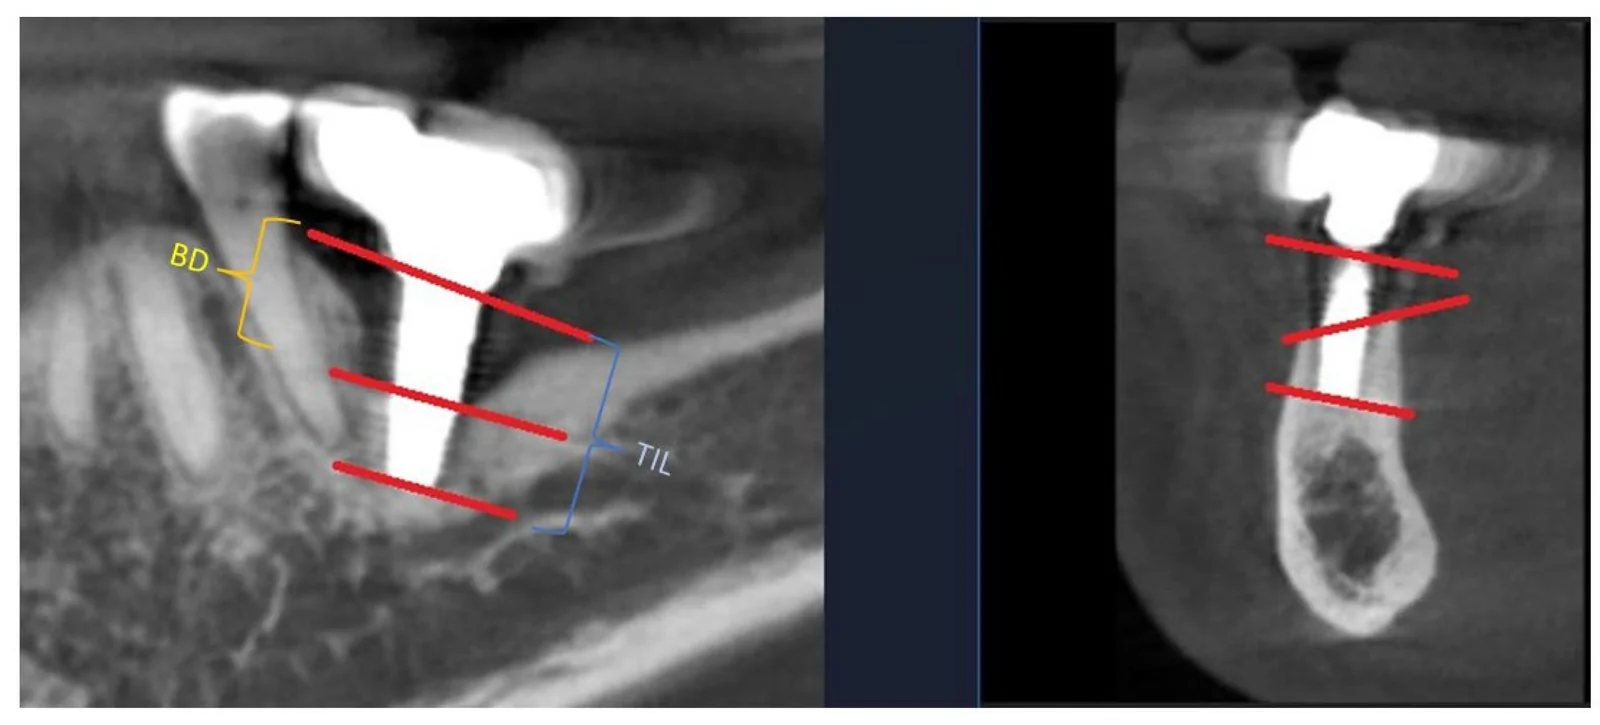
CBCT-based assessment of peri-implant bone loss.
